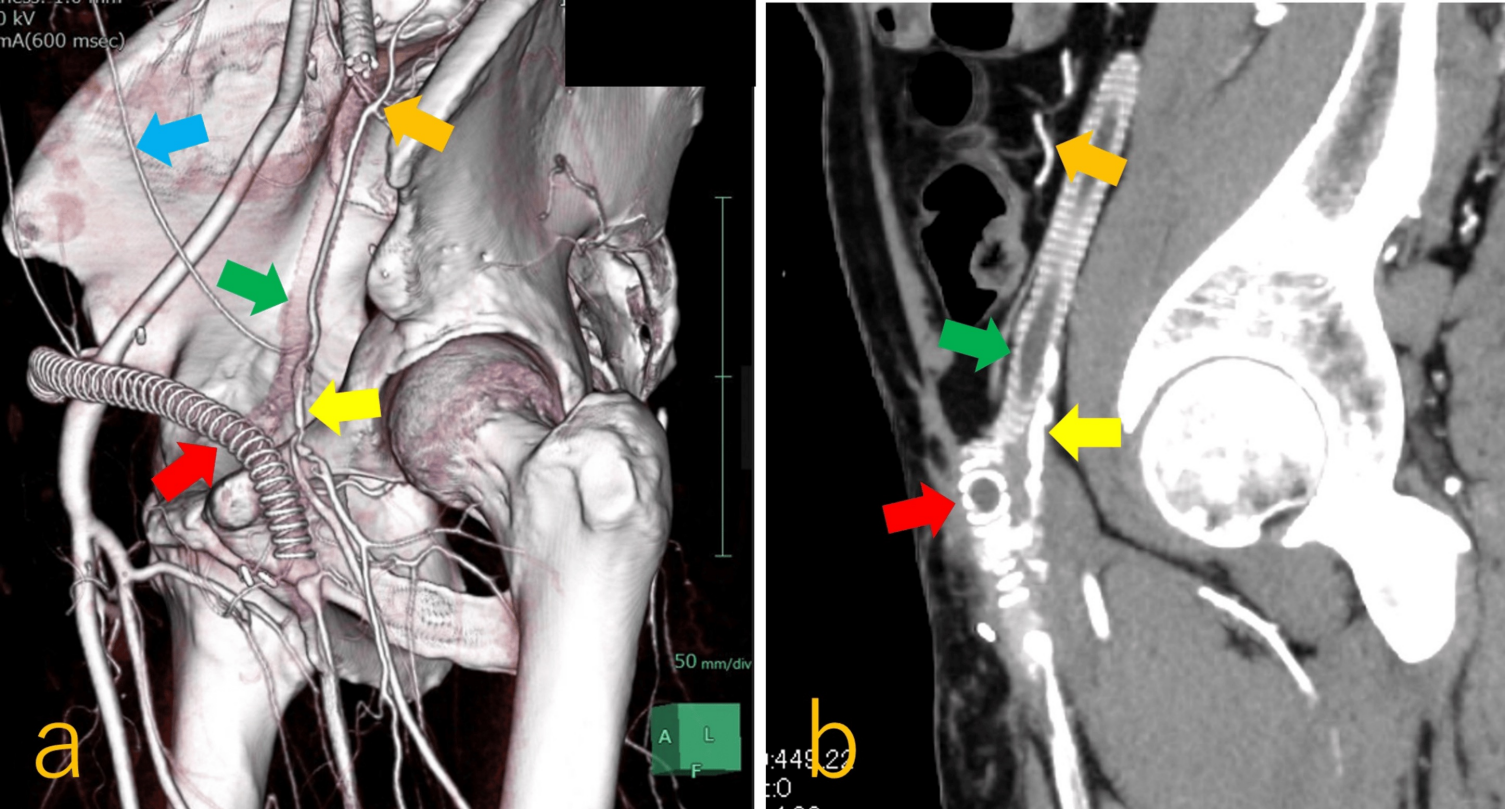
Preoperative CT finding of obturator foramen crossover bypass grafting a: three-dimensional CT showing the two occluded prosthetic bypasses: the green arrow indicates a terminal aorta–left CFA bypass, whose distal anastomosis was detached, and the red arrow indicates a right EIA–left SFA crossover bypass, the blue arrow marks the inferior epigastric artery, the orange arrow indicates the iliac circumflex artery, and the yellow arrow identifies the CFA; b: the left CFA (yellow arrow) and SFA were narrow and shaggy, the CFA was suspected to be compressed between the two prosthetic bypasses (green and red arrows), the caput femoris when the hip joint was flexed, and the orange arrow indicates the iliac circumflex artery. EIA: external iliac artery; SFA: superficial femoral artery; CFA: common femoral artery
